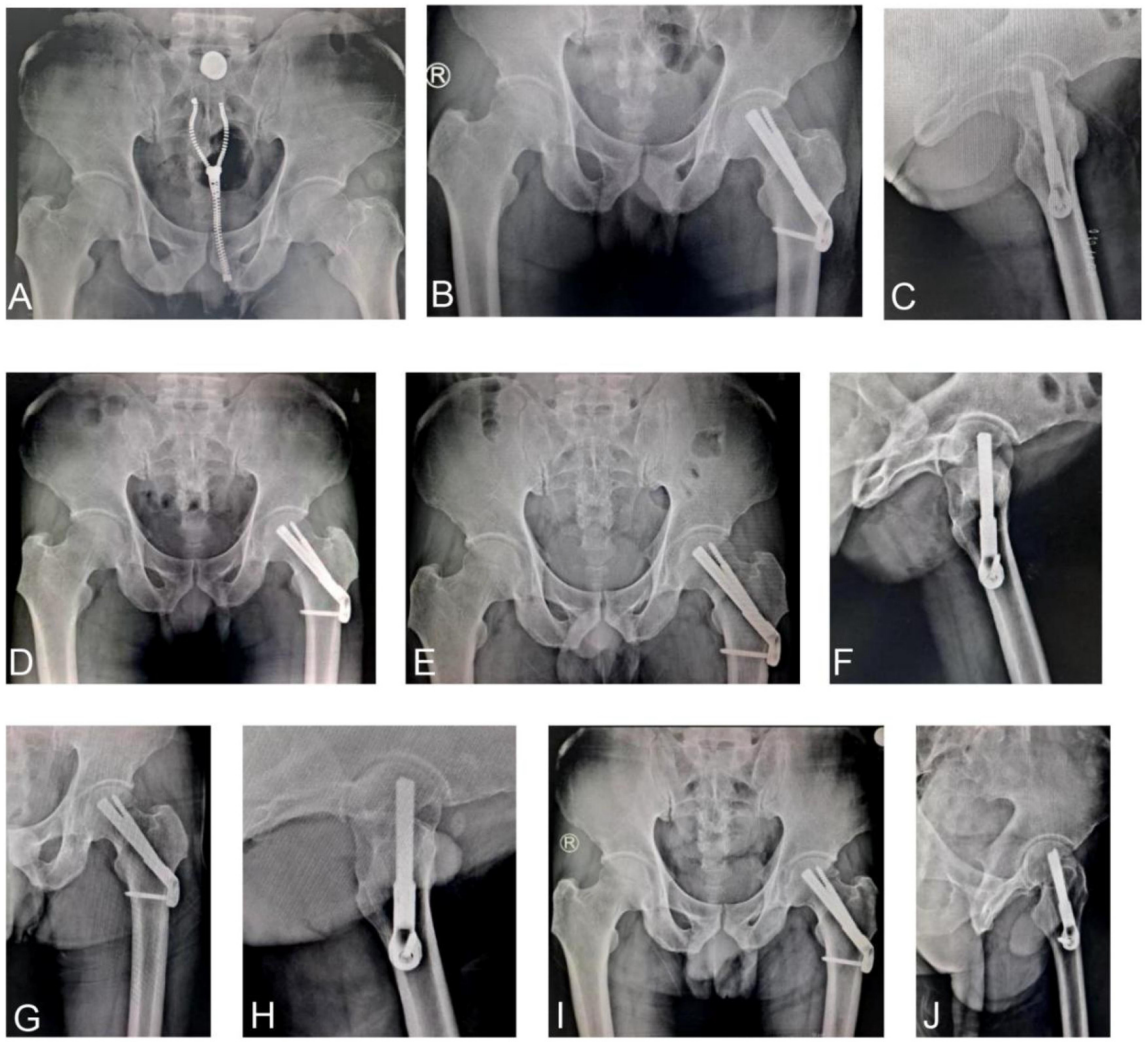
A 56-year-old patient sustained a Pauwels type III FNF following a fall and underwent FNS 24 h after injury. (A), Pre-surgical pelvic x-ray in AP view; (B,C), AP (B) and lateral (C) views on day 1 postoperatively; (D), Anteroposterior x-ray at 1 month postoperatively; (E,F), AP (E) and lateral (F) x-rays obtained 3 months after surgery, showing a blurred fracture line; (G,H), AP (G) and lateral (H) x-rays at 6 months postoperatively, revealing complete fracture healing; (I,J), AP (I) and lateral (J) x-rays at 12 months postoperatively.
